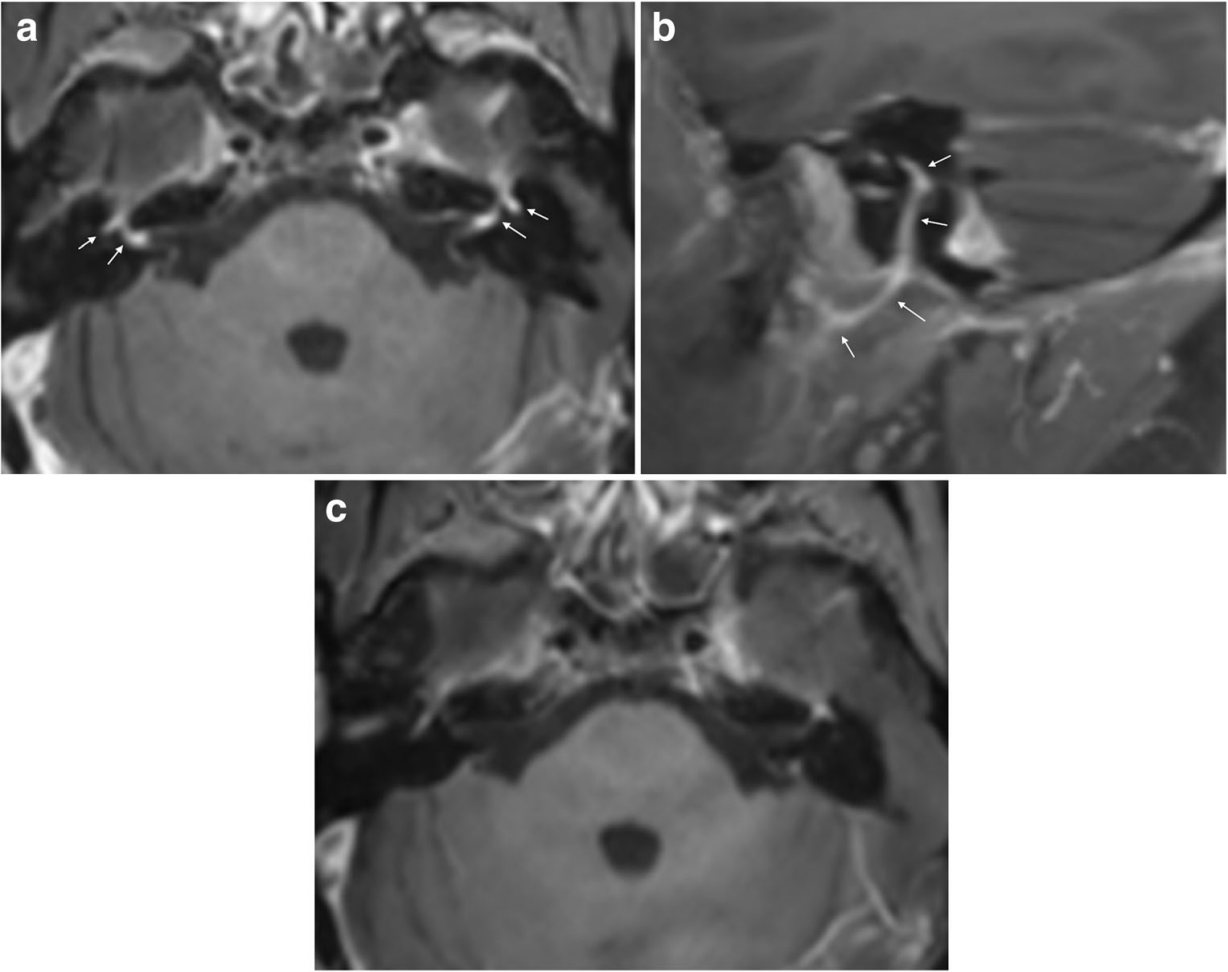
Facial neuritis in LNB with typical disease course in a 55-year-old female. She suffered from back pain radiating to chest, arms, head and neck, especially intense at night time, and after 2 weeks she also developed bilateral facial palsy. CSF analysis showed 594 cells/mm^3^ (normal range 0-5/mm^3^) and production of *Bb* specific IgG and IgM antibodies. Axial post-contrast fat suppressed T1-weighted image (a) showed intense enhancement in the distal intracanalicular, labyrinthine and geniculate ganglion segments of both facial nerves (arrows point to these structures which are mentioned from medial to lateral). 3 mm sagittal reformat (b) on the right side demonstrated the enhancement involving the tympanic and mastoid segment, as well as the parotid segment with the temporofacial and cervicofacial branches at the pes anserinus included (arrows point to these structures which are mentioned from cranial to caudal). Axial post-contrast fat suppressed T1-weighted image showed no abnormal enhancement of facial nerves 6 months after the treatment (c)
